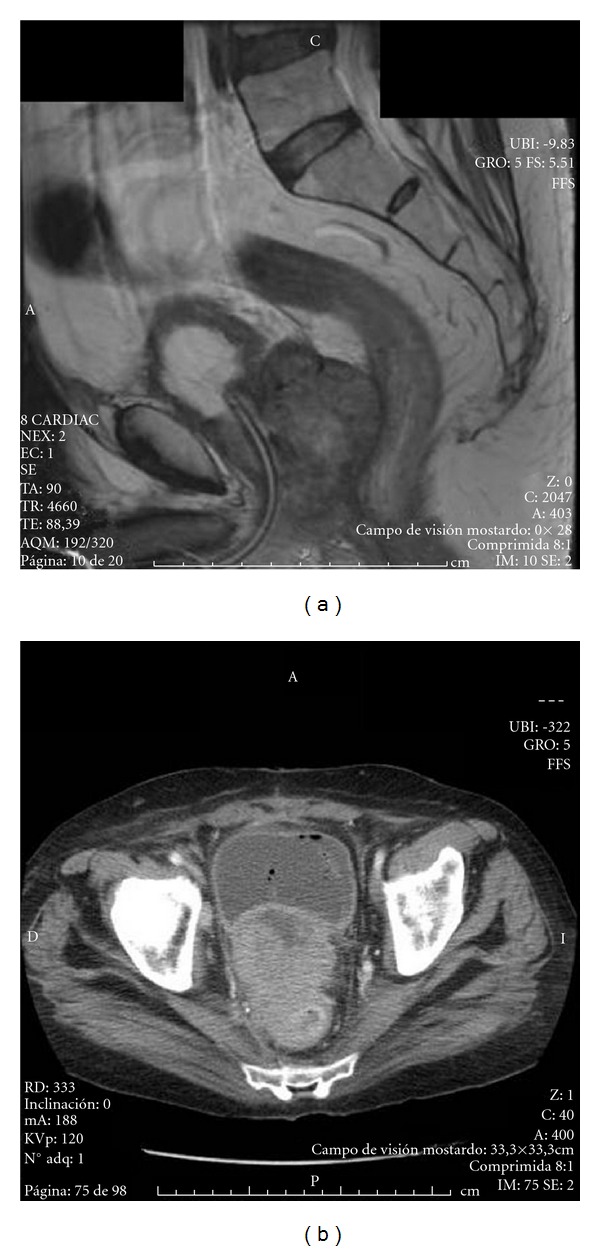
(a) Pelvic MRI considered suspicious for extracapsular extension of the tumour. (b) Pelvic TC scan showed tumour with extents hypodense zones, consistent with necrosis.
